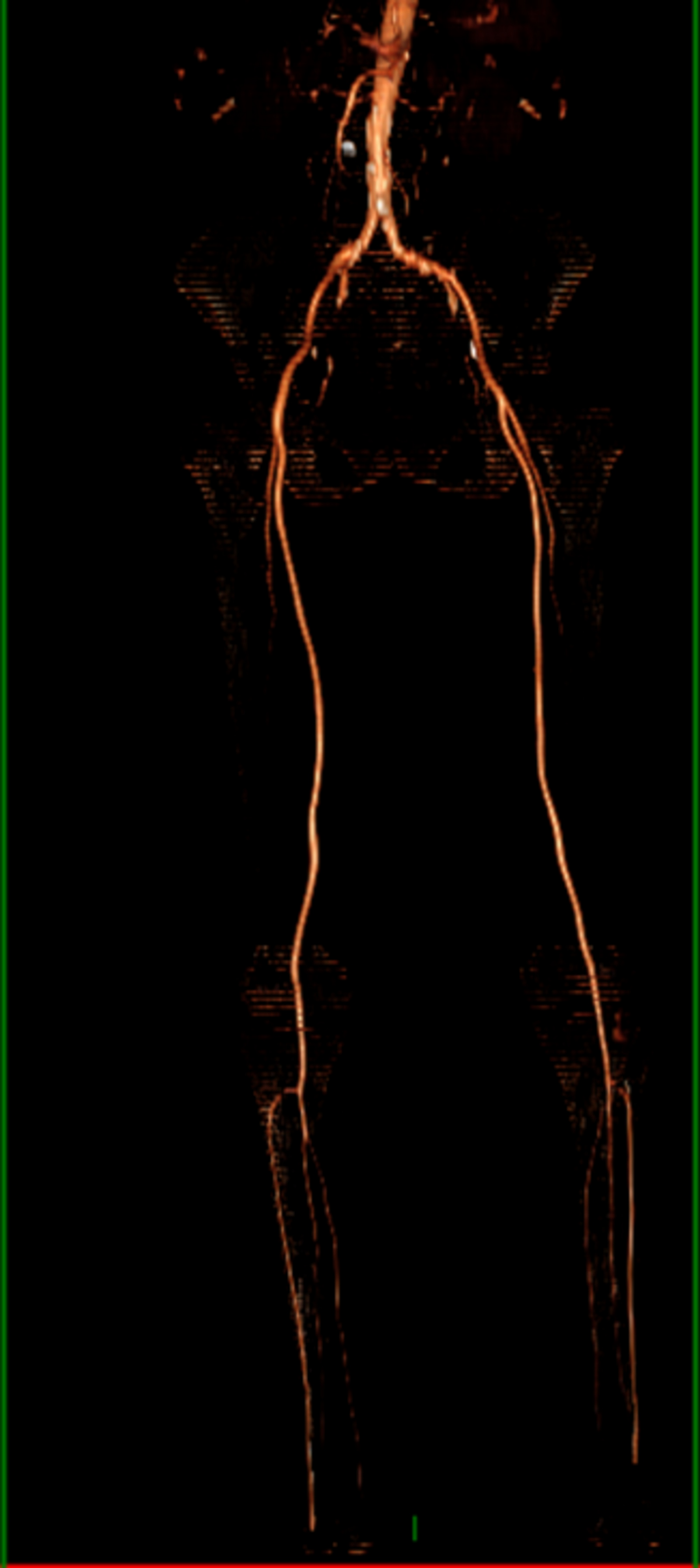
CT angiography of the aorta and its branches with no significant stenosis CT: computed tomography
